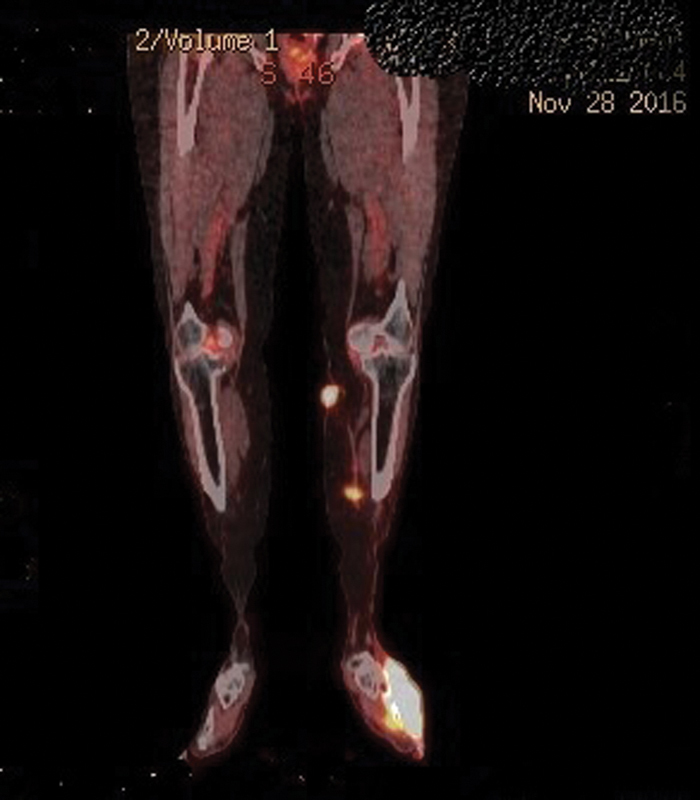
FDG-PET/CT showed a primary lesion at the dorsum of the left foot (SUV: 13.6), extensor muscles, and subcutaneous fat tissue. CT, computed tomography; FDG, fluorodeoxyglucose; PET, positron emission tomography.
